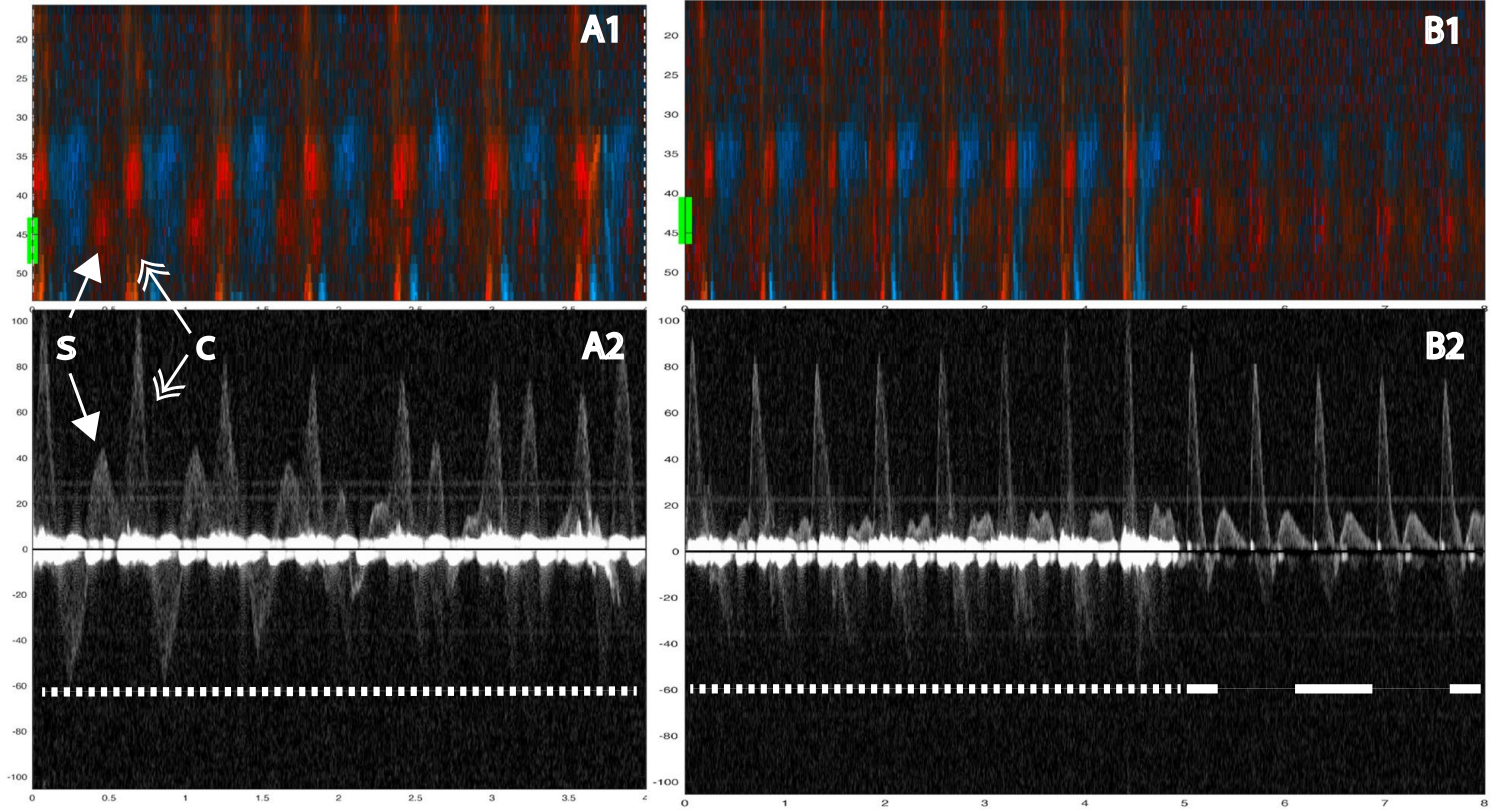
Two examples showing evidence of spontaneous circulation. In A1 (color M-mode) during manual compressions at 100/min, one sees spontaneous circulation appearing as red signals at a depth of about 40 mm (yellow line at the margin). The corresponding Doppler spectrum (A2) from the chosen depth of 40 mm shows spontaneous velocity signals interspersed between (and out of phase with) the compression-generated spectra. In B1 (color M-mode) during manual compressions at 100/ min, first one sees compression (tissue) artifacts across the entire depth obscuring the spontaneous signals, which mainly become evident during the circulatory check. In B2 the spontaneous velocity signals are immediately visible at the circulatory check. Below both panels, a narrow-dashed line indicates ongoing chest compressions, and a wide-dashed line indicates a circulatory check B. The two double arrows: the single-tip arrows indicate spontaneous circulation (S), and the double-tip arrows indicate chest compression (C). Velocities are in cm/s
